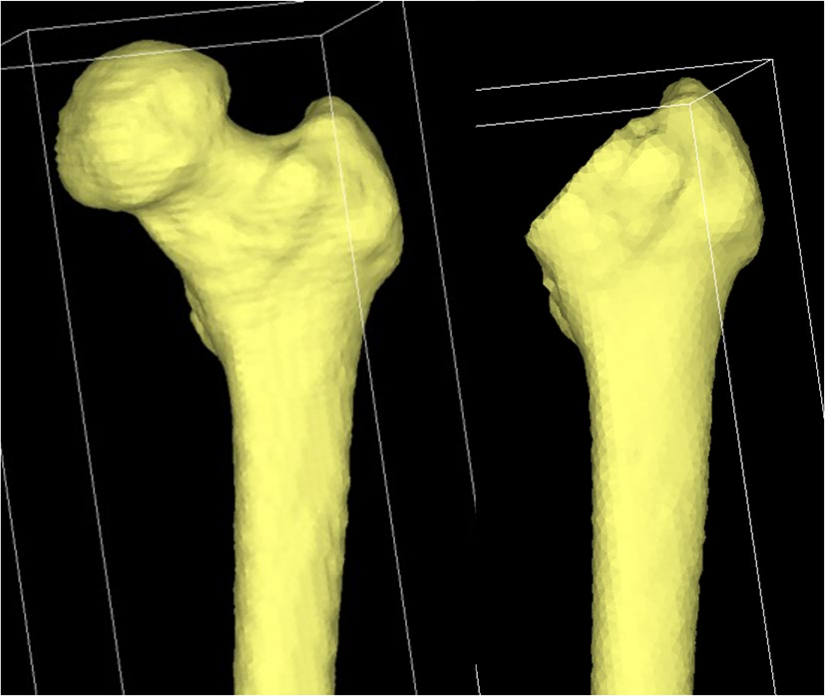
3D bone model. The DICOM data of the preoperative femur CT were read to extract the contour of the femur and create a 3D bone model using tetrahedral elements. The femur was osteotomized approximately 10 mm from the lesser trochanter
